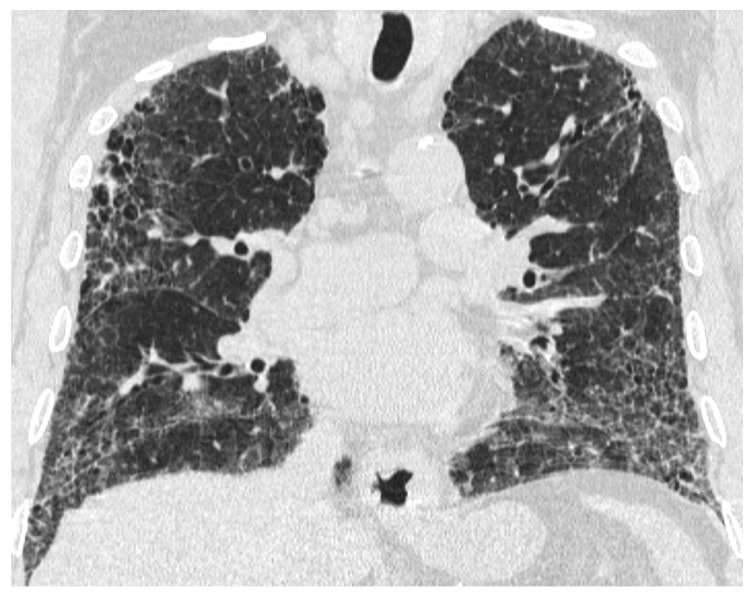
An axial CT scan of a patient with IPF/UIP. HRCT abnormalities are mostly diffuse without craniocaudal predominance.
